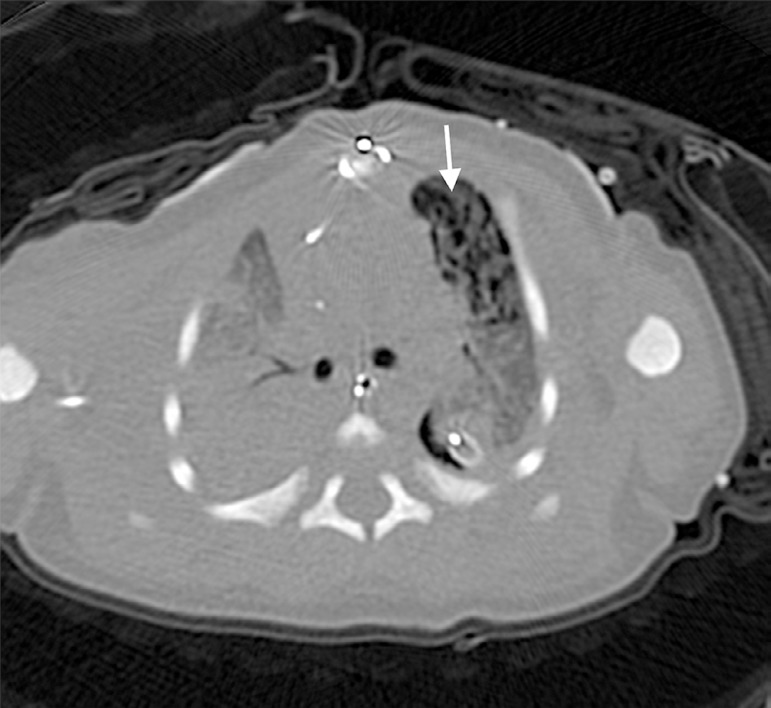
Transverse computed tomography displaying left upper lobe pulmonary
